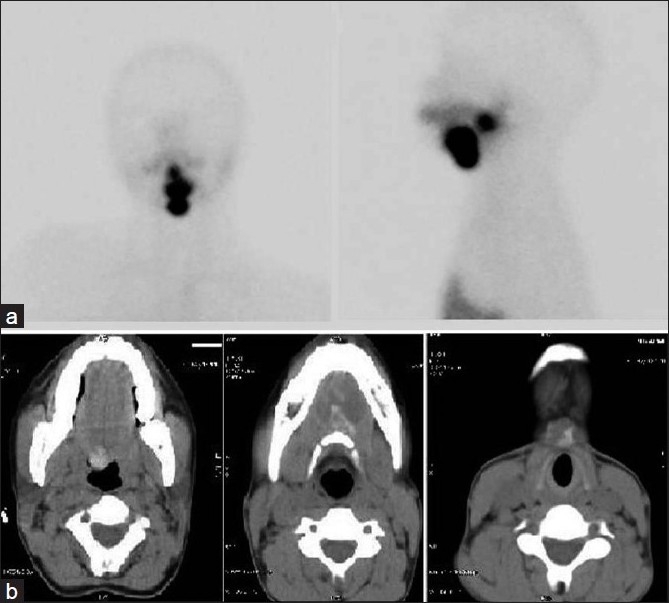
(a, b) Thyroid scan and CT image (axial section at different levels) showing triple ectopia - sublingual, suprahyoid, and subhyoid - with absence of normal thyroid
